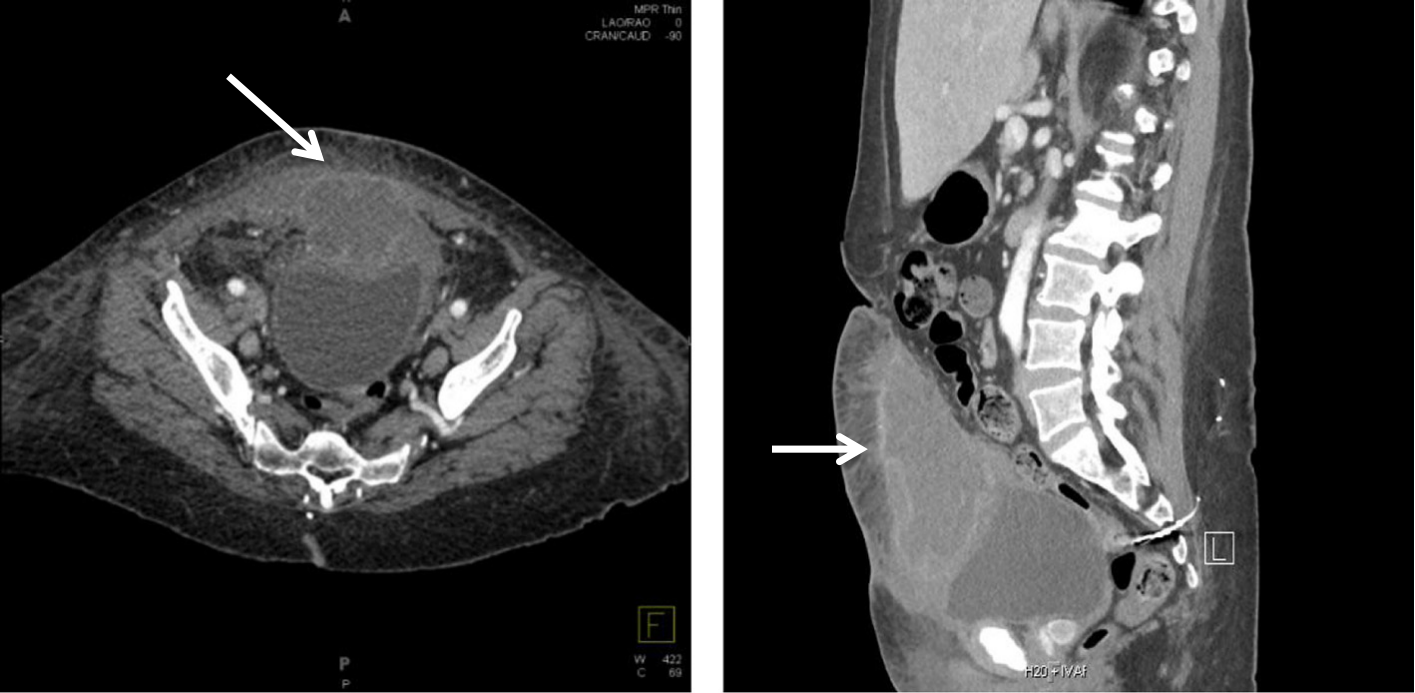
CT scan of the abdomen and pelvis, axial (left) and sagittal (right) views. The mass can be seen extending anteriorly and superiorly from the dome of the bladder (arrows).
